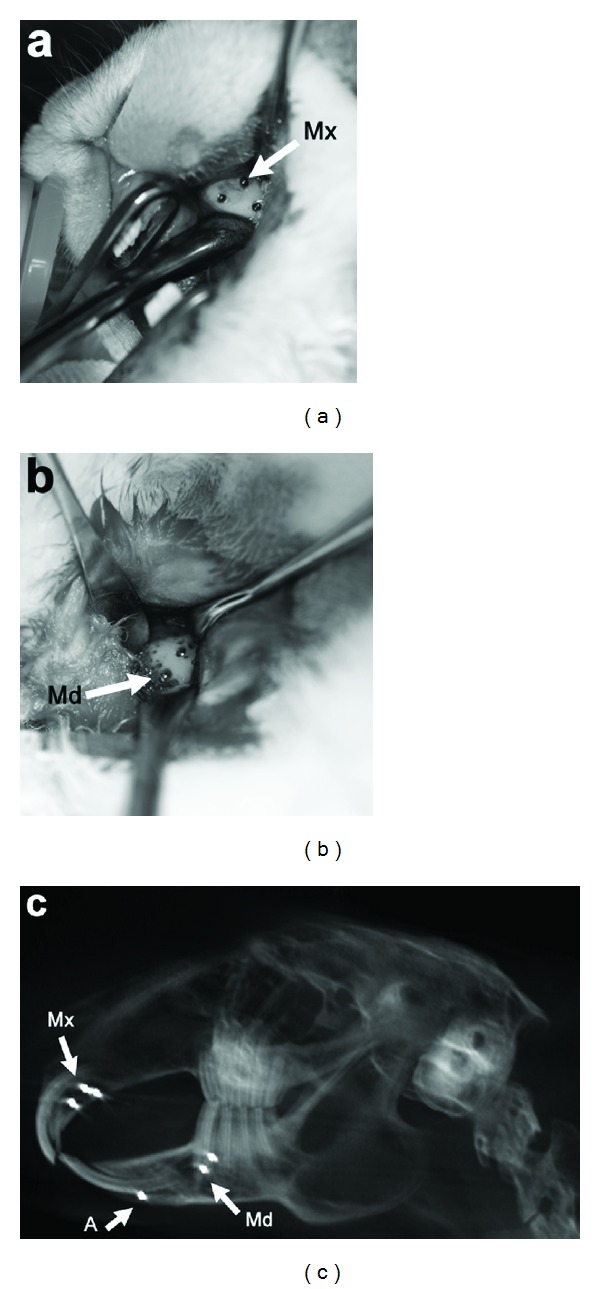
Surgical photographs and baseline radiograph showing placement of the metallic fiduciary markers. (a) Placement of 1 mm diameter tantalum spheres into the incisive bone of the maxilla (white arrow, Mx); (b) placement of spheres into the body of the mandible (white arrow, Md); and (c) baseline lateral cephalometric radiograph generated from cone-beam computerised tomographic volume, showing implants in the maxilla (Mx), body of the mandible (Md), and anterior mandible (A).
